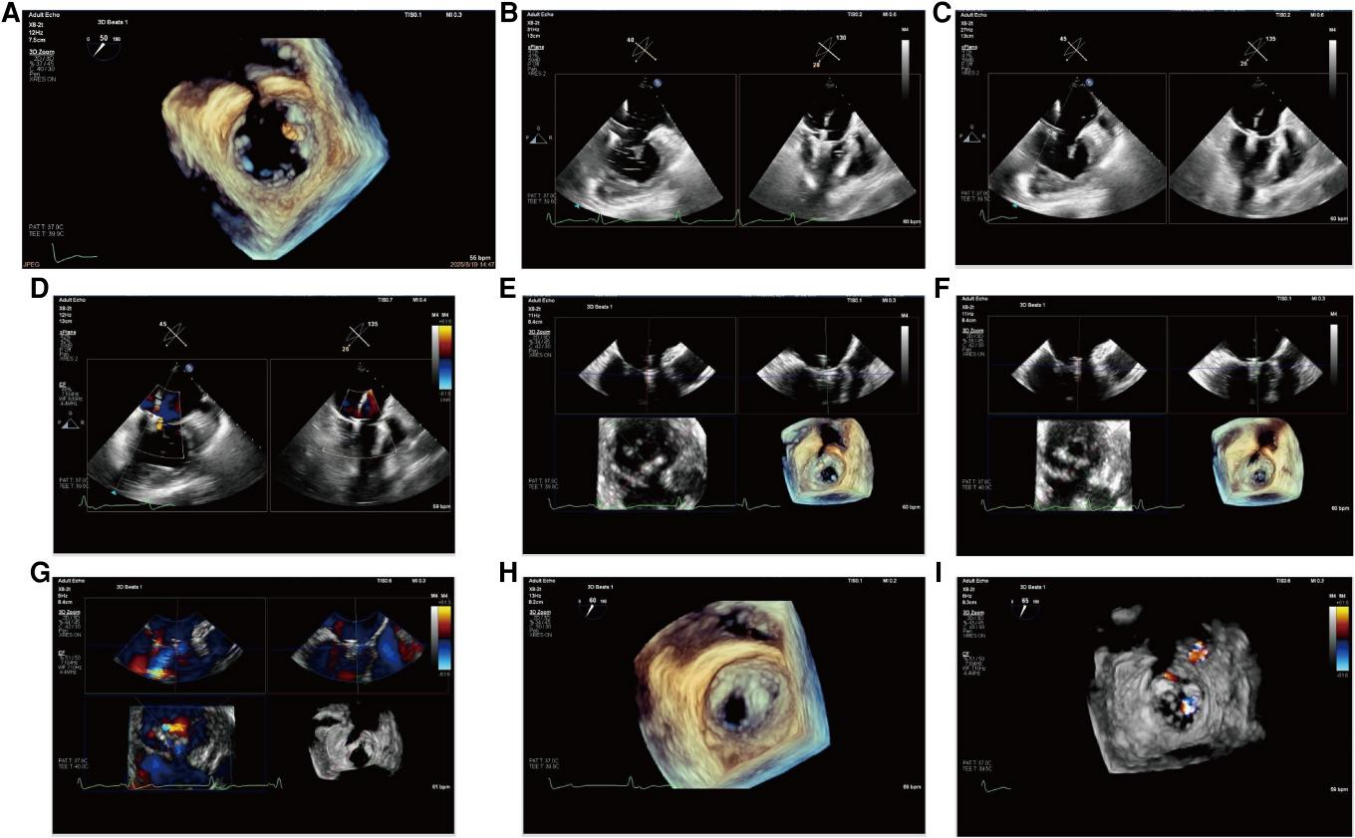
Stepwise transcatheter edge-to-edge repair of the systemic atrioventricular valve. (*A*) Orientation of the first clip perpendicular to the posterior–septal coaptation line under echocardiographic guidance. (*B*) Grasping of the posterior and septal leaflets. (*C–D*) Clip closure and stable leaflet capture leading to a marked reduction of posterior–septal regurgitation. (*E*) Positioning and orientation of the second clip perpendicular to the anterior–septal coaptation line, followed by leaflet grasping. (F–G) Closure of the second clip, with anterior–septal regurgitation reduced to trace severity. (H–I) Post-release three-dimensional echocardiography demonstrating stable tissue bridges created by both clips, with only mild to moderate (grade 1–2+) residual systemic atrioventricular valve regurgitation.
